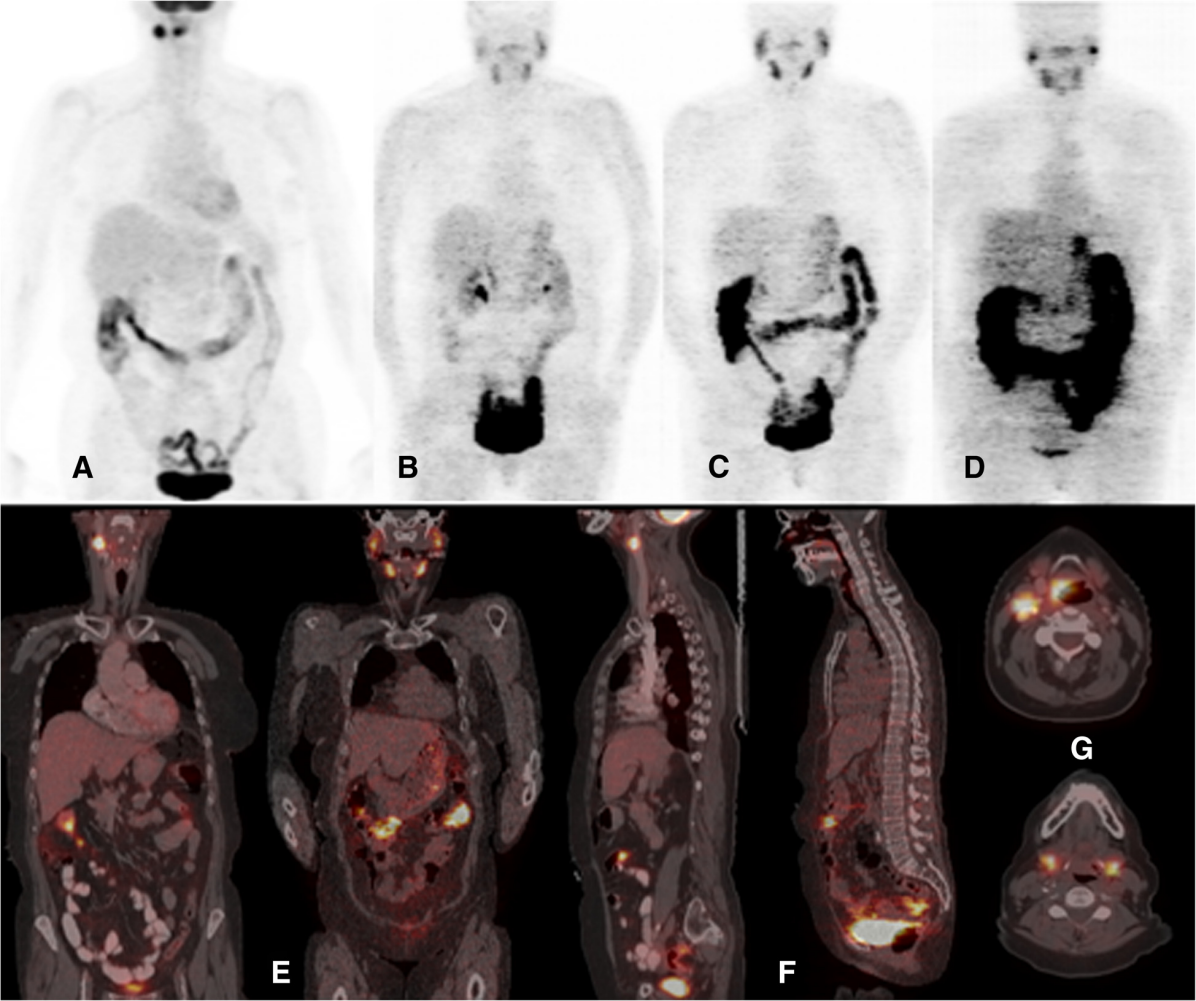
PET/CT images of ^18^F-FDG and ^124^I-IAZGP in a head and neck cancer patient. (A to D) Coronal maximum intensity projection PET images of ^18^F-FDG acquired 3 days before ^124^I-IAZGP administration (A) and ^124^I-IAZGP acquired at 3 h (B), 6 h (C), and 27 h (D) post-administration in a patient with head and neck cancer. (E to G) Cross-sectional fused PET/CT images of the same patient comparing ^18^ F-FDG (E and F left, G top) with ^124^I-IAZGP at 6 h (E and F right, G bottom). No evidence of ^124^I-IAZGP uptake is seen in either the FDG-avid supraglottic larynx cancer or the approximately 2-cm lymph node. The clearance of ^124^I-IAZGP is similar to that seen in Figure 1, with a symmetric distribution of physiologic ^124^I in the salivary glands. Note that image intensities are not directly comparable and that the ^18^F-FDG image was acquired in the radiotherapy treatment position with the patient in a facial mask.
